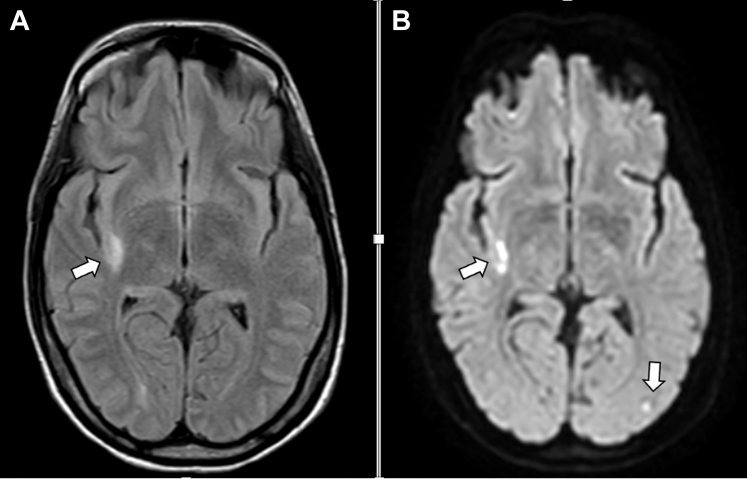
Maternal Brain Magnetic Resonance Imaging Brain magnetic resonance imaging of the patient showing acute stroke lesions due to cerebral embolism in different vascular territories. Embolic lesions are visible on axial (A) fluid attenuated inversion recovery images and (B) diffusion-weighted imaging.
